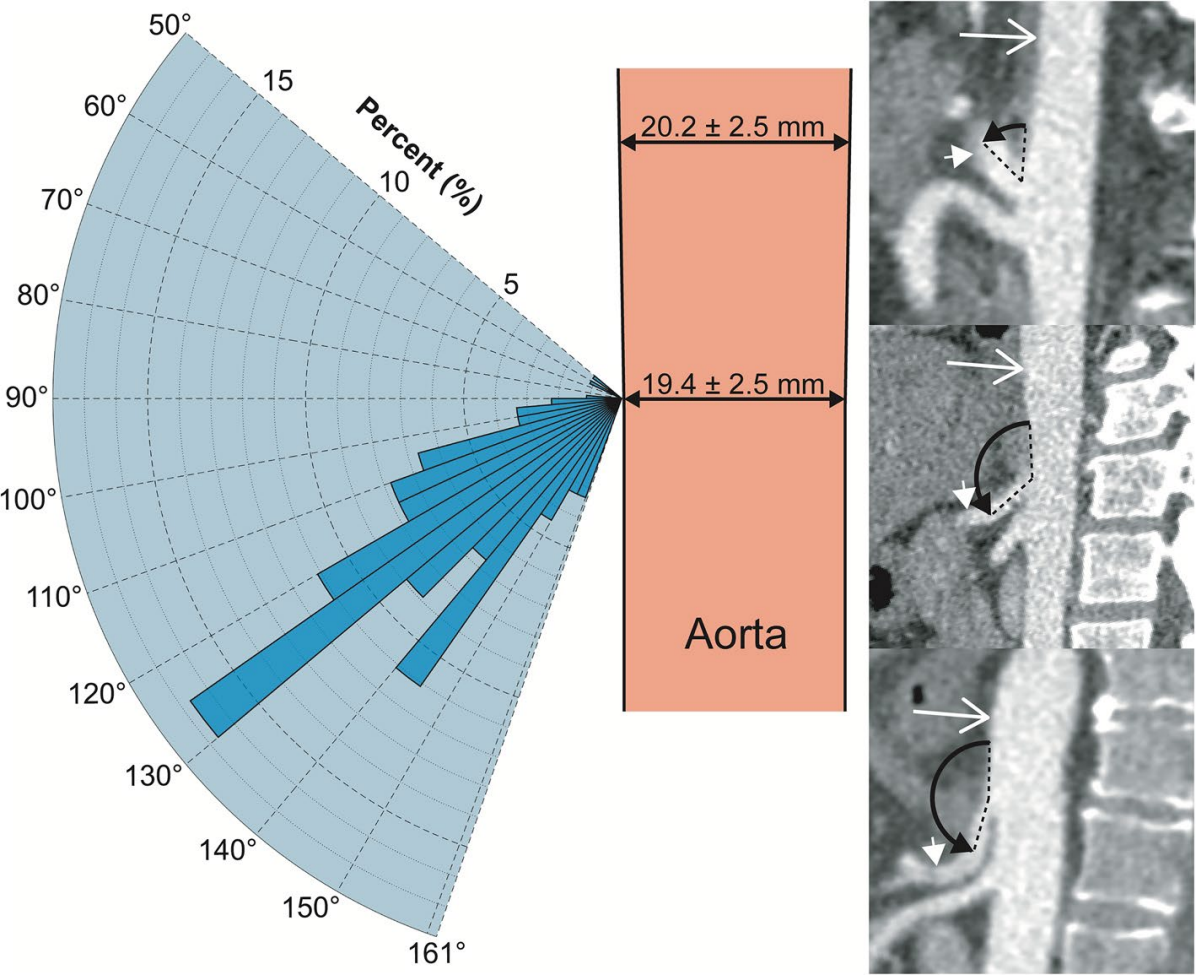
Angle of the origin of the coeliac trunk from the aorta in the para-sagittal plane. Histogram of the angle of origin of coeliac trunk from the aorta in the para-sagittal plane plotted as a percentage of participants. The minimum and maximum angle is represented by the radial range of the graph (50 to 161°). The anterior-posterior dimensions of the aorta at the level of the coeliac trunk are also included. Inset CT images show examples and calculations for the coeliac trunk para-sagittal angle from participants in the (top to bottom) lowest, median and highest decile. Long white arrow, aorta; short white arrow, coeliac trunk; black arrow, measured angle
